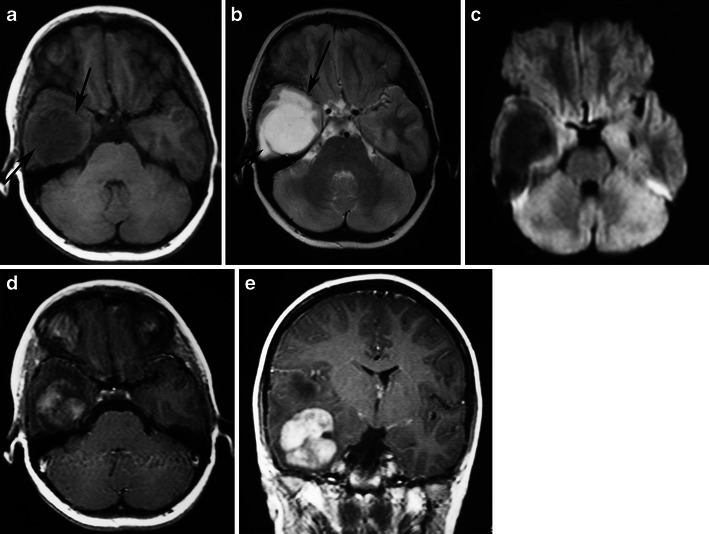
Supratentorial pilocytic astrocytoma with solid enhancement and vasogenic oedema. a An axial T1-weighted image shows a well-circumscribed, hypointense cerebral mass (arrows). b An axial T2-weighted image shows a hyperintense mass. Note the vasogenic oedema around the mass (arrows). c The lesion exhibits low signal on diffusion-weighted imaging. Contrast-enhanced axial (d) and (e) T1-weighted images demonstrate inhomogeneous enhancement of the mass
